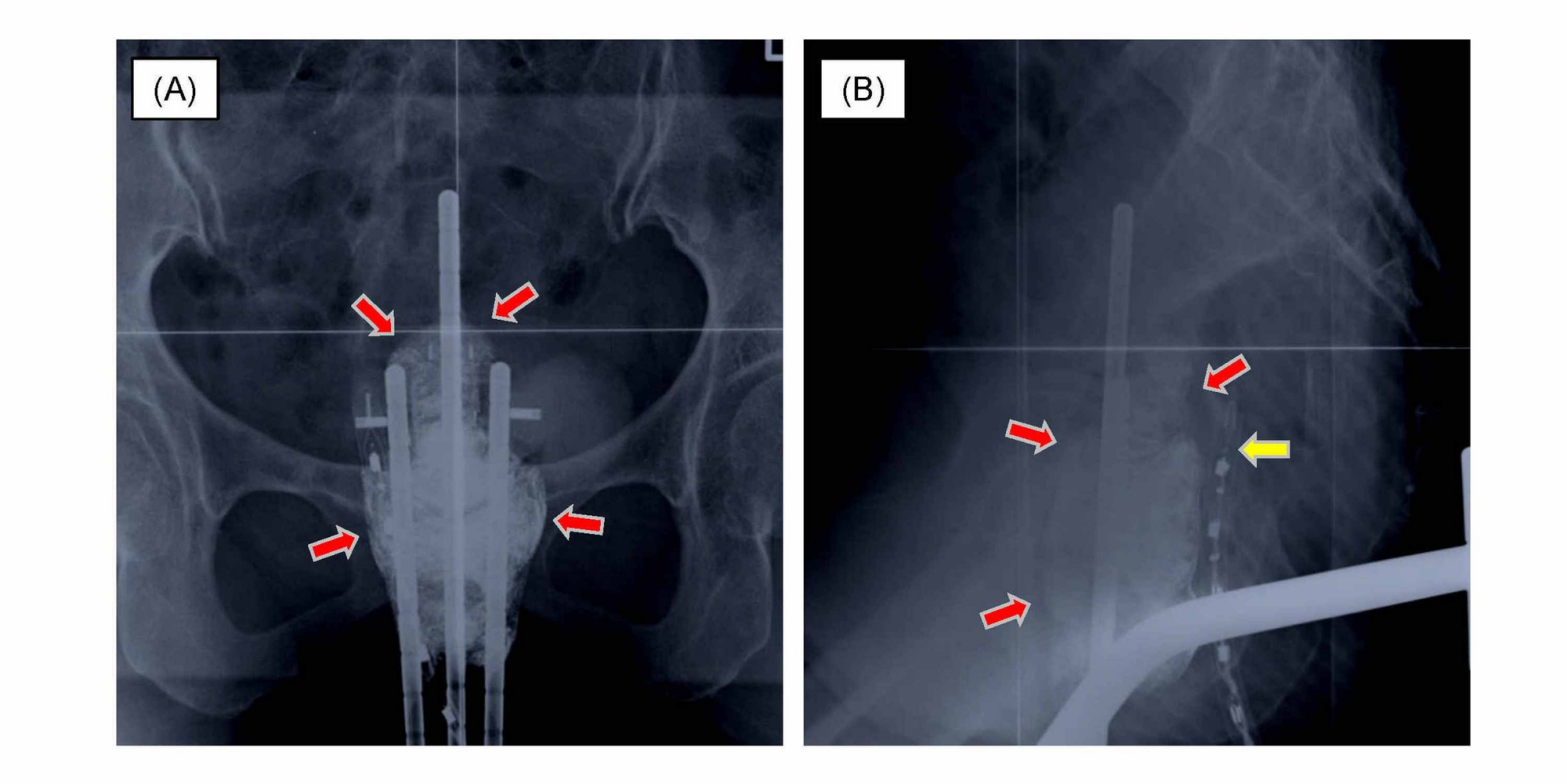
Two-way radiographs of two-dimensional intracavitary brachytherapy. Inserted packs between the uterus and rectum or bladder (A, B, red arrows), and the dosimeter to the rectum (B, yellow arrow).
